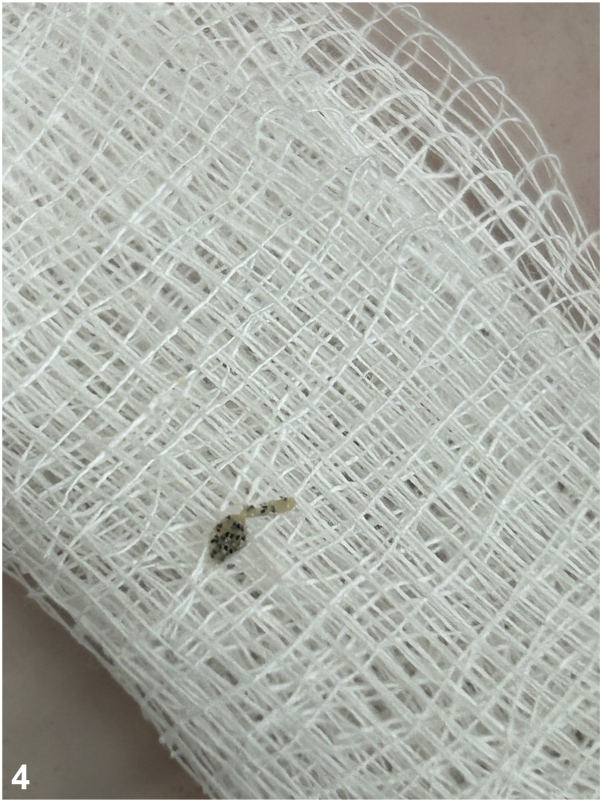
Question 1: Considering the clinical presentation, dermoscopy, and ultrasonographic findings, what is the diagnosis?
